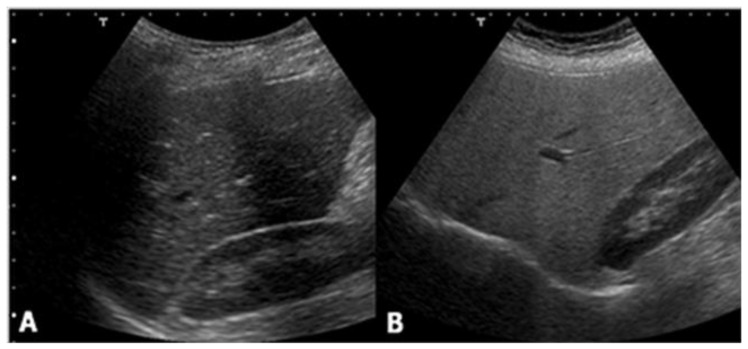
Ultrasound shows no difference between (A) the liver and right kidney cortex echogenicity, while (B) it demonstrates a hyperechoic liver compared with the kidney parenchyma.
